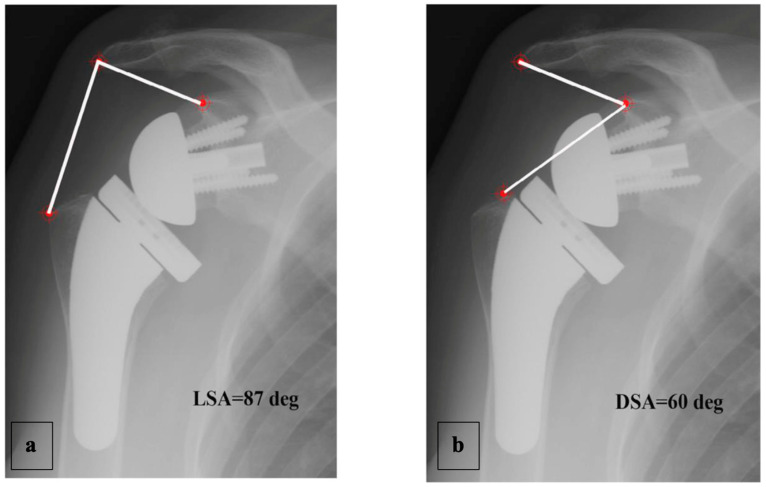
Example of LSA (a) and DSA (b) measured on an AP radiograph in neutral shoulder rotation, as described by Boutsiadis et al. [12].
